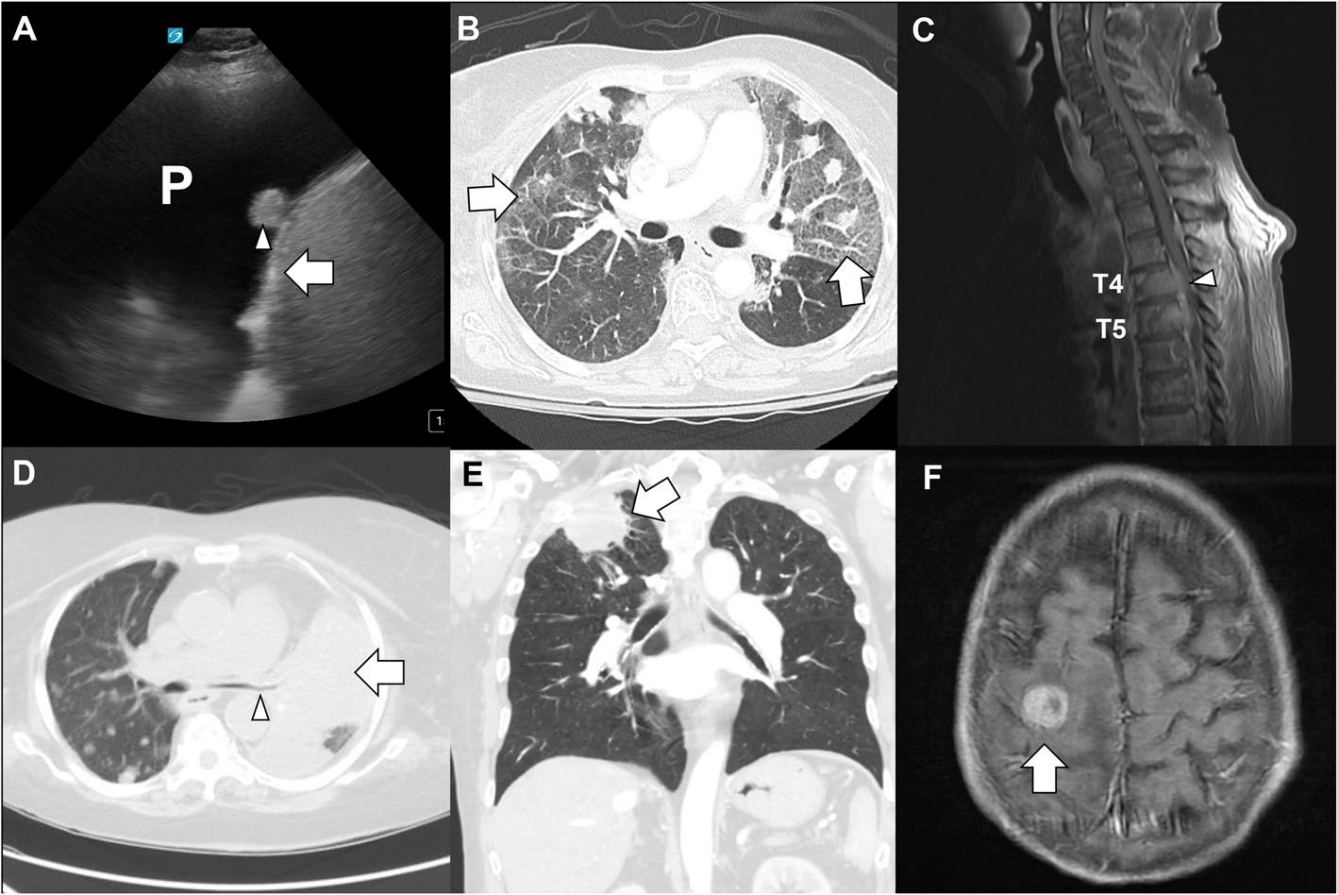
Manifestations of disease. A Ultrasound image of suspected malignant pleural effusion (P) with nodule (arrowhead) on the diaphragm (arrow). B Lung cancer on immune checkpoint inhibitor with multiple nodules and ground glass infiltrates (arrows, left > right) concerning immune-mediated pneumonitis. C Extensive small cell lung cancer with metastatic disease to spine (T4, T5) and extension of epidural tumor (arrowhead). D Left lung mass (arrow) with extrinsic compression (arrowhead) of the left mainstem bronchus. E Elderly aged man with a right upper lobe lung mass (arrow) in the apex consistent with a Pancoast tumor (mass that originates in the superior sulcus of the lung apex which may present atypically with shoulder pain, Horner syndrome, and superior vena cava syndrome) presenting with right shoulder pain and cough. F Middle-aged man with metastatic poorly differentiated lung cancer presents to the emergency center after fall. Imaging reveals a 1.8-cm right posterior frontal mass (arrow) with local edema
